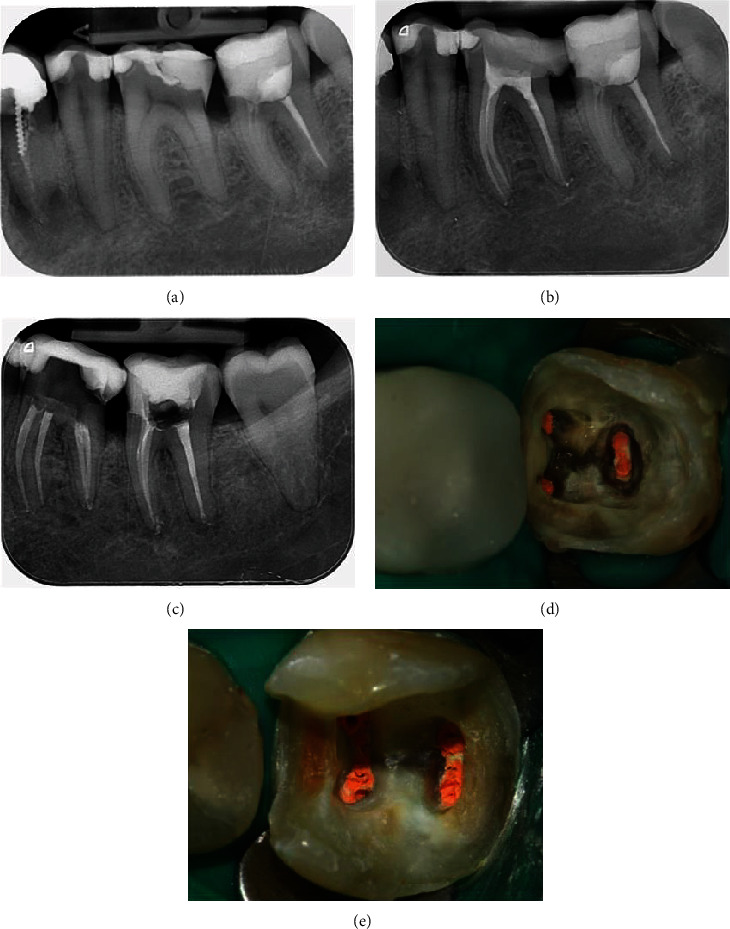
Preoperative images: radiograph images of tooth 36 (a) and 37 (b) prior to endodontic treatment and postendodontic treatment (c) and clinical view of tooth 37 (d) and 36 (e).
